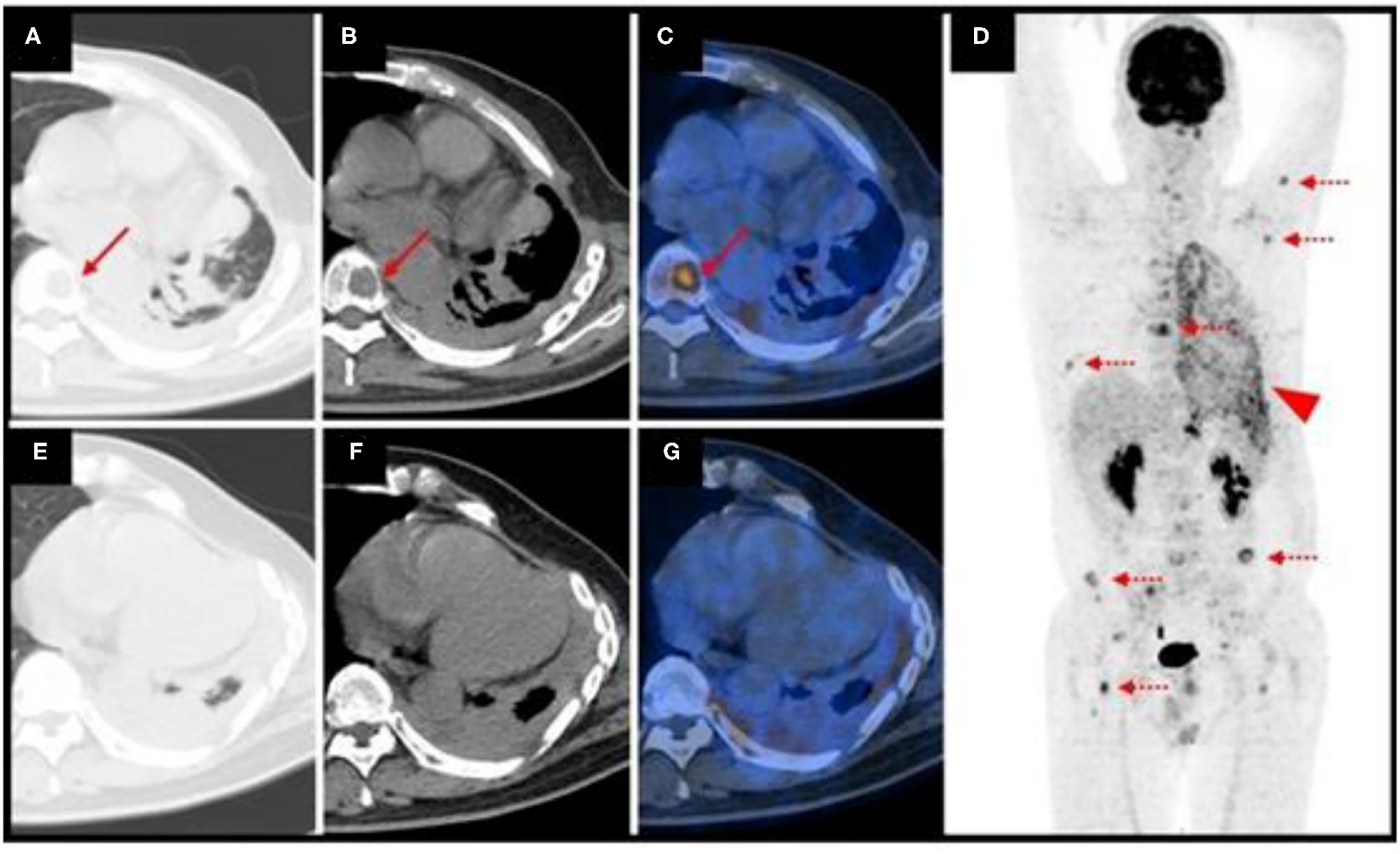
Repeat position emission tomography/computed tomography (PET/CT) imaging showed multiple foci of abnormal activity in the left lung and skeleton. The arrows denote a vertebral body lesion. Dotted arrows indicate multiple bone lesions. The arrowhead indicates intense FDG uptake in the left chest (A,B,E,F) axial CT; (C,G), axial fused PET/CT; (D) maximum intensity projection.
